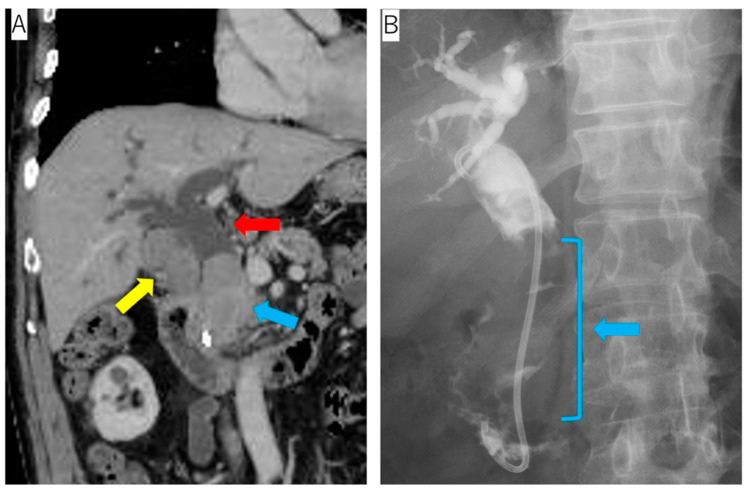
Radiological evaluation. (A) Contrast-enhanced CT revealed an ischemic tumor of the gallbladder (yellow arrow) and common bile duct (blue arrow) as well as common bile duct dilation (red arrow). (B) ERCP revealed an expansive tumor in the common bile duct.
